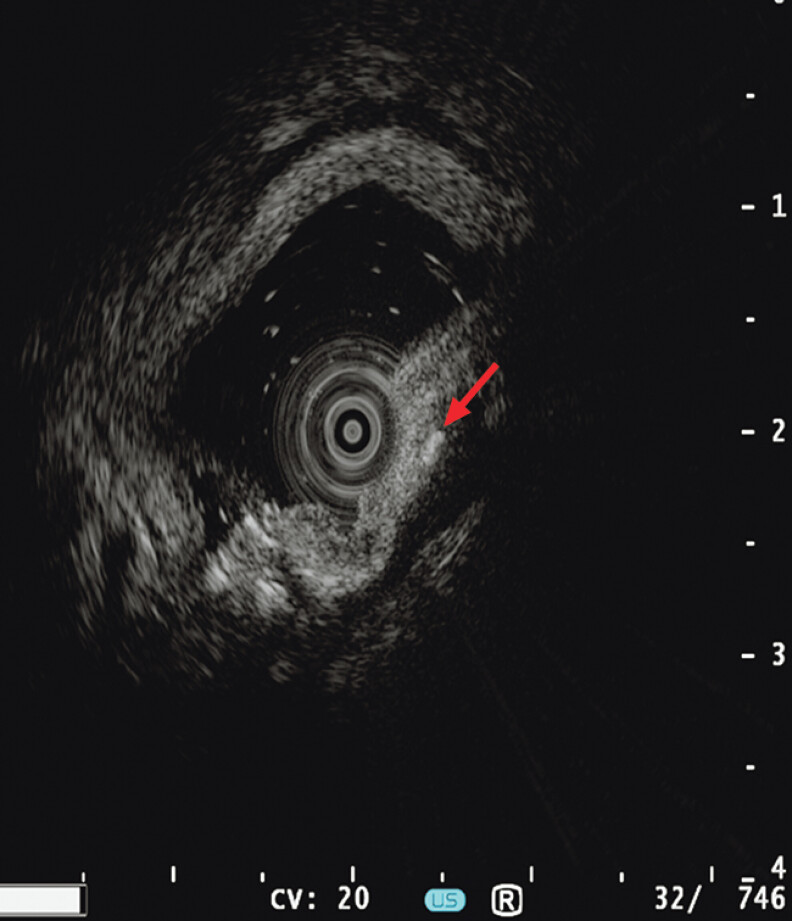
Ultrasound gastroscopy showed a hyperechoic space with a cross-section of approximately 2 mm × 2 mm in the submucosal layer and muscularis propria of the duodenal bulb mass.
